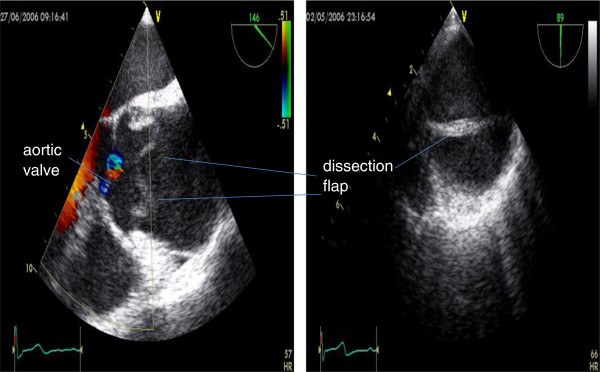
a) Dilated aortic root on transoesophageal echo with evidence of dissection flap b) Transaxial view of aortic dissection.
